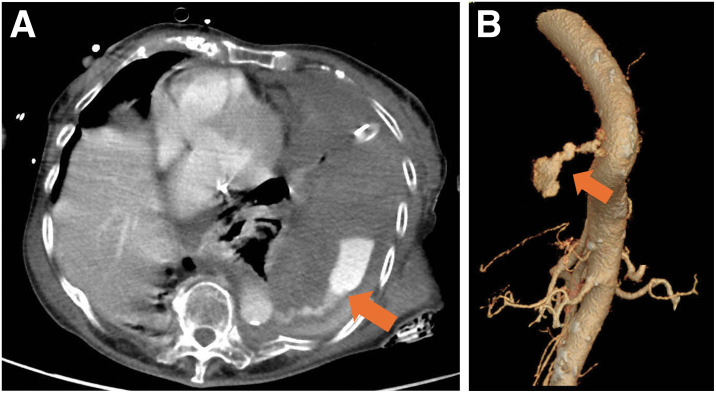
Contrast-enhanced CT after clinical deterioration. (A) Axial contrast-enhanced image demonstrating a massive left hemothorax with active contrast extravasation in the left posterior hemithorax. (B) 3D VR reconstruction demonstrating active contrast extravasation and its spatial relationship to the displaced posterior rib fractures. The arrows indicate active contrast extravasation. VR, volume-rendered
